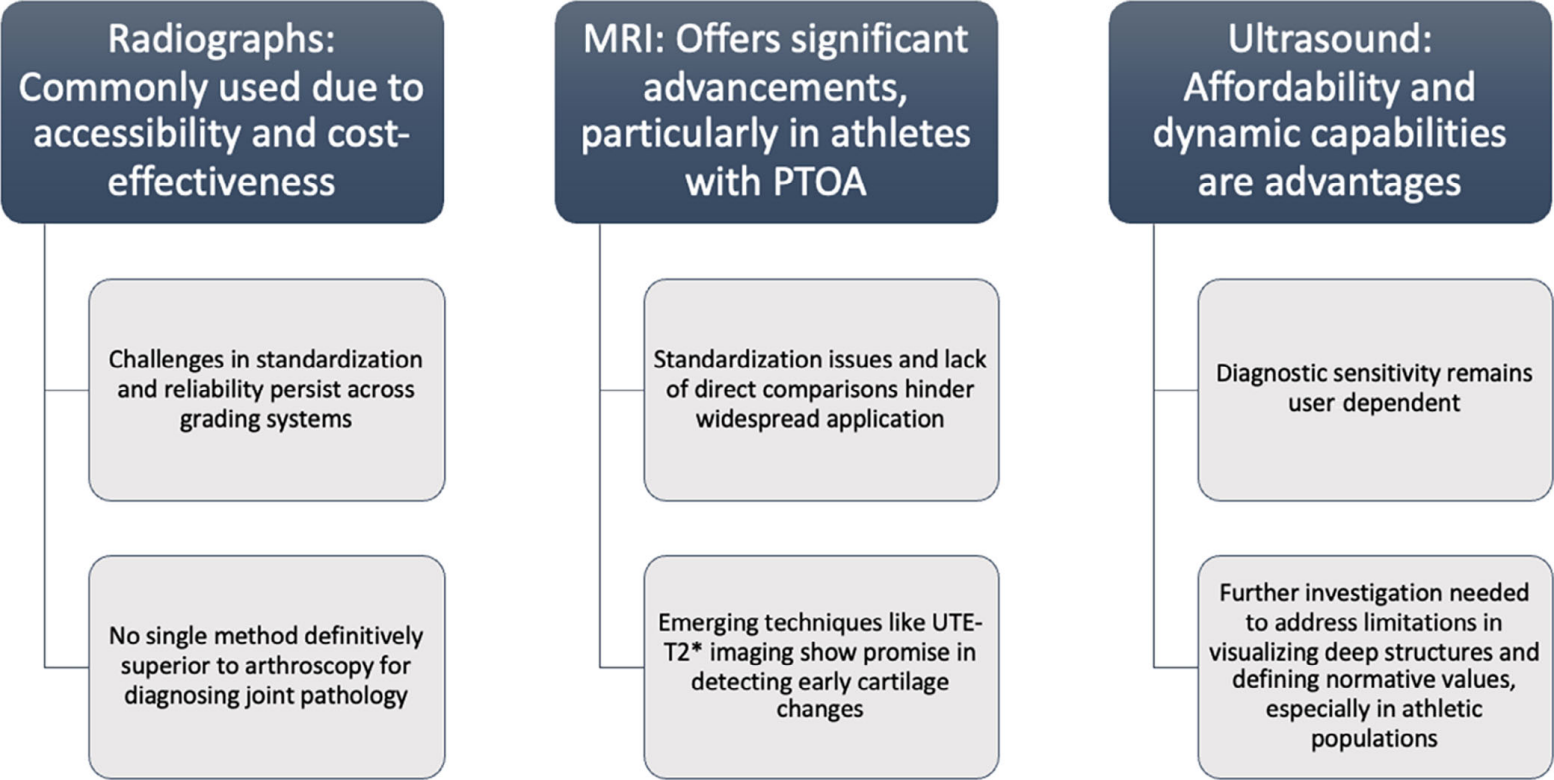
Summary of imaging modalities in osteoarthritis and post‐traumatic arthritis diagnosis. MRI, magnetic resonance imaging; PTOA, posttraumatic arthritis; UTE‐T2*, ultrashort echo‐time enhanced T2*.
